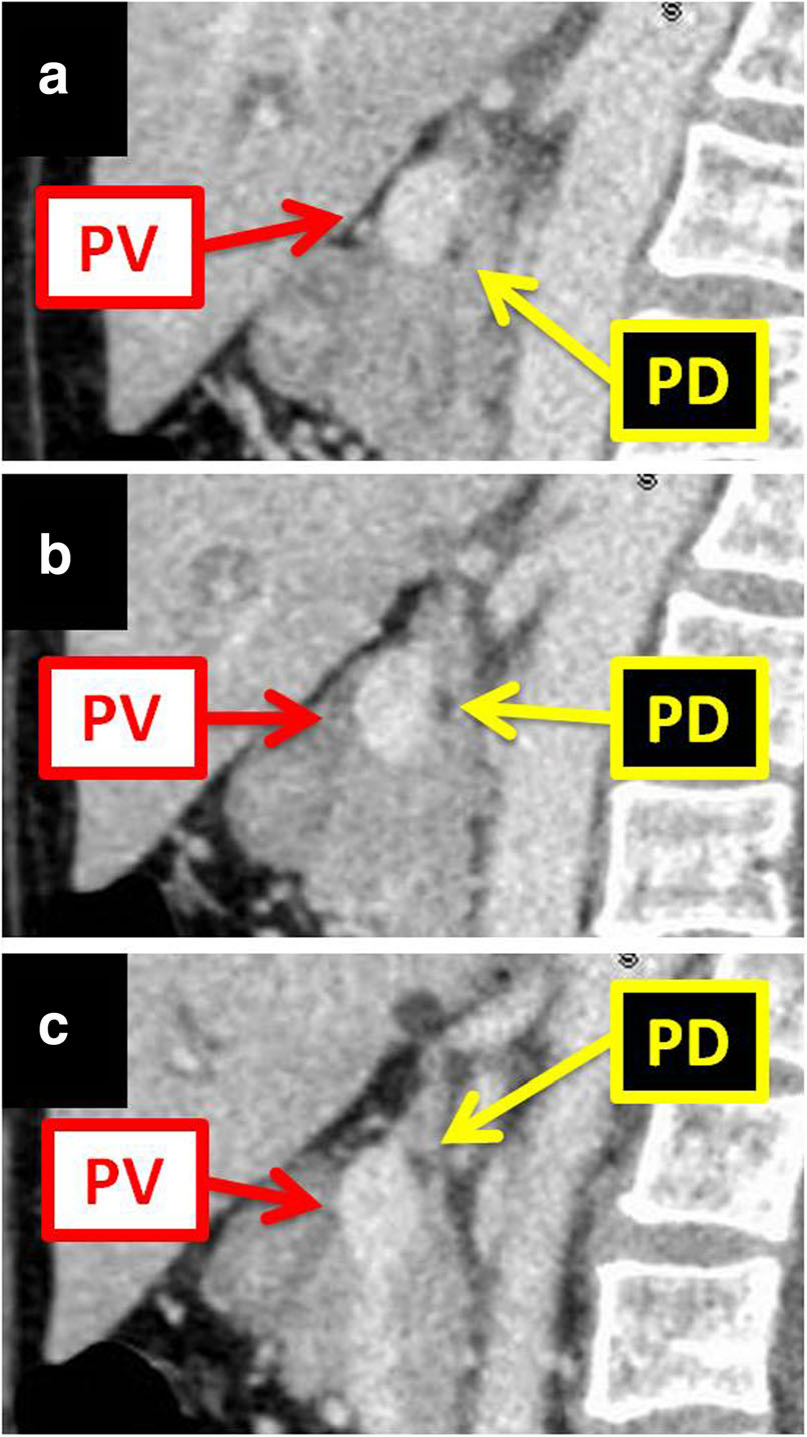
Sagittal images of computed tomography of the abdomen. The images show the pancreatic duct coursing posterior to the portal vein. Three sequential cuts shown: a–c right to left. *PD* pancreatic duct, *PV* portal vein
